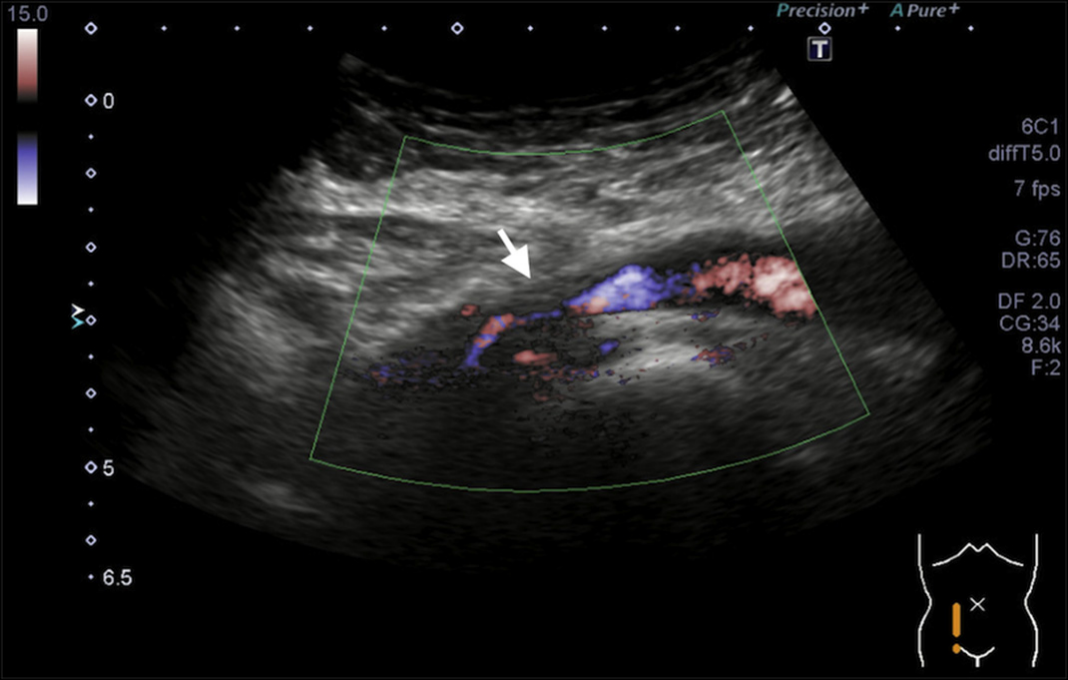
Ultrasonography of the Right Femoral Vein Stenosis The ultrasound scan indicated suspected right femoral vein stenosis (arrow).
